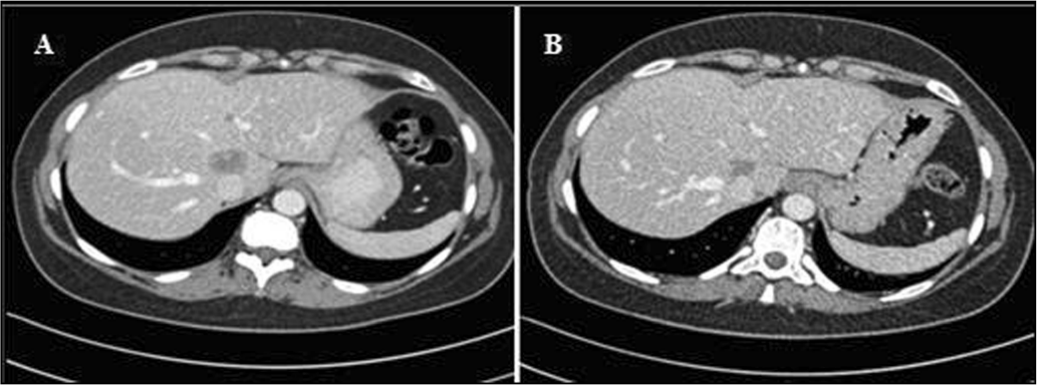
Tumor response after the first three cycles of NKTR-102. A: Baseline abdominal computed tomography scan showing liver metastasis. B: Abdominal computed tomography scan showing a partial response after three cycles of pegylated irinotecan NKTR-102.
